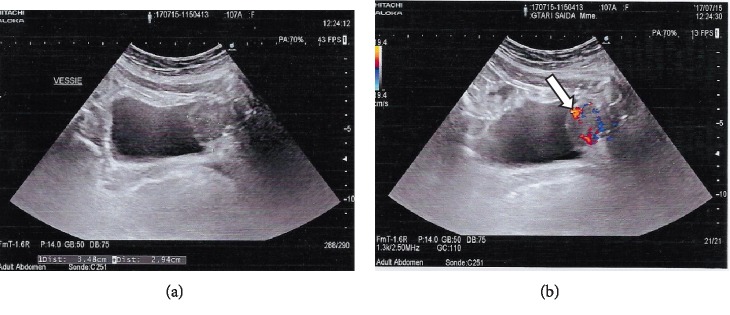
(a) Sagittal gray-scale ultrasound image of the urinary bladder shows a heterogeneous 40 mm mass along the anterior wall of the bladder. (b) Sagittal color Doppler ultrasound image demonstrates marked flow within the bladder mass (arrow).
